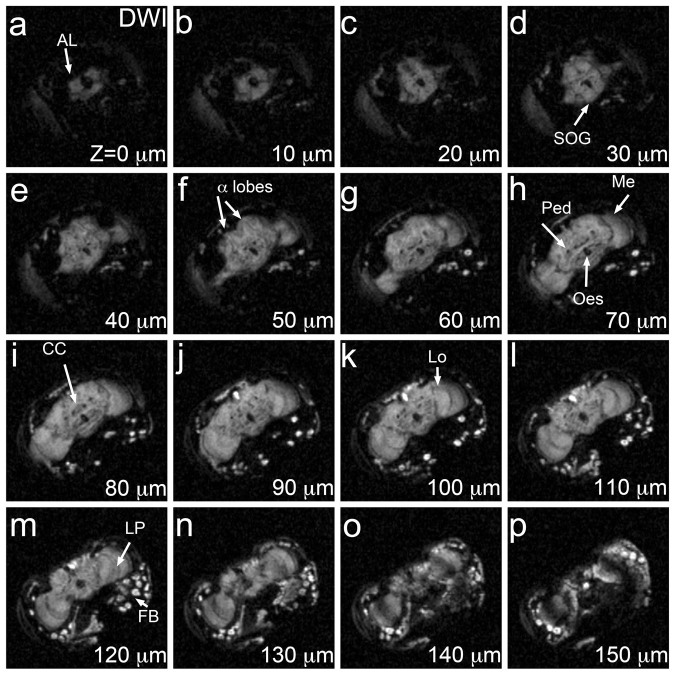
3D DWI at 10 μm^3^ resolution. 3D DW MRM of the same *Drosophila* head. The DW MRM series is slightly darker
